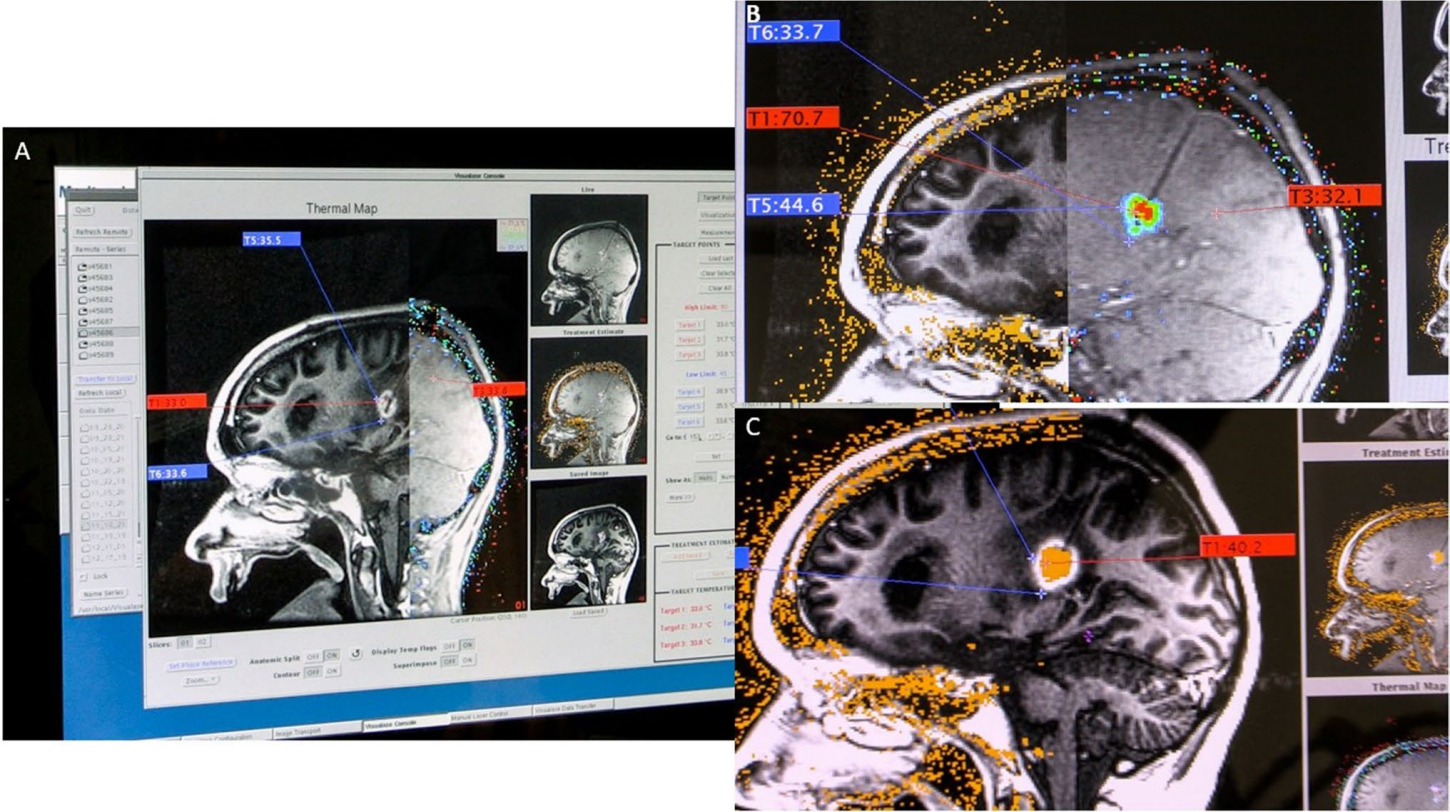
LITT ablation procedure A. High-temperature markers (< 90°) are set in the vicinity of the catheter tip to protect it from overheating and low-temperature markers (< 45–50°) close to critical areas to be protected (in this case the motor fiber tracts). Irrigation is started to ensure cooling of the catheter during treatment. B. A low-energy test dose is delivered to confirm heat distribution in the intended area. The energy is increased for the ablation itself and the tissue temperature changes are followed on the console as color changes on the imaging, while the lesional damage estimate is calculated by the system and monitored continuously on the console. C Contrast-enhanced T1 3D volume MRI (FSPGR) is performed after the ablation and compared to the damage map from the ablation. A contrast-enhancing peripheral ring (in addition to the contrast enhancing tumor) on the post-ablational MRI scan marks the border of the ablated volume which is seen to correspond well with the estimated damage map (orange)
